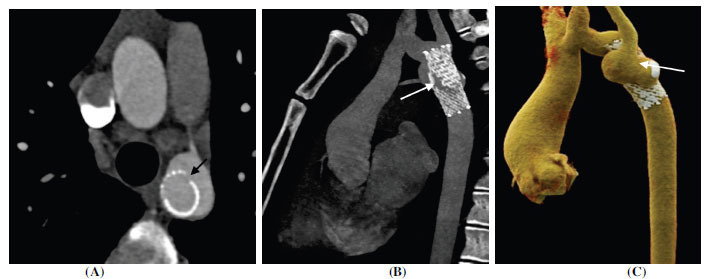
Pseudoaneurysm in aortic coarctation post stent placement. (A) Axial contrast-enhanced computed tomographic angiography image showing metallic stent at the site of previous aortic narrowing. The stent lumen appears patent. The stent integrity is lost at focal places (arrow) with contained leakage of contrast on the anterior and lateral aspects. (B) Sagittal contrast-enhanced computed tomographic angiography image and (C) Volume rendered technique images showing loss of stent integrity with pseudoaneurysm formation around it.
